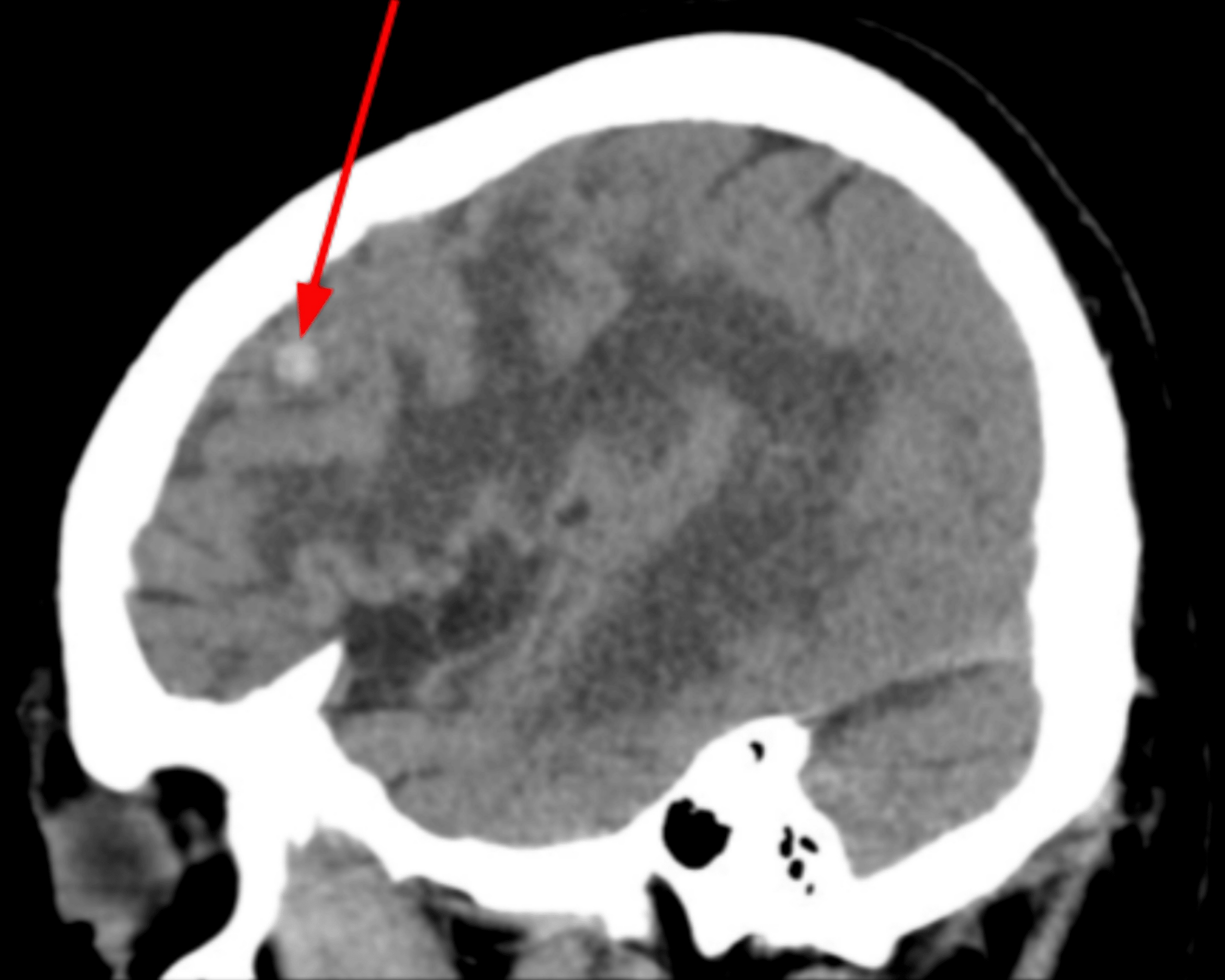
Brain MRI (October 2024) showing stability of metastases
